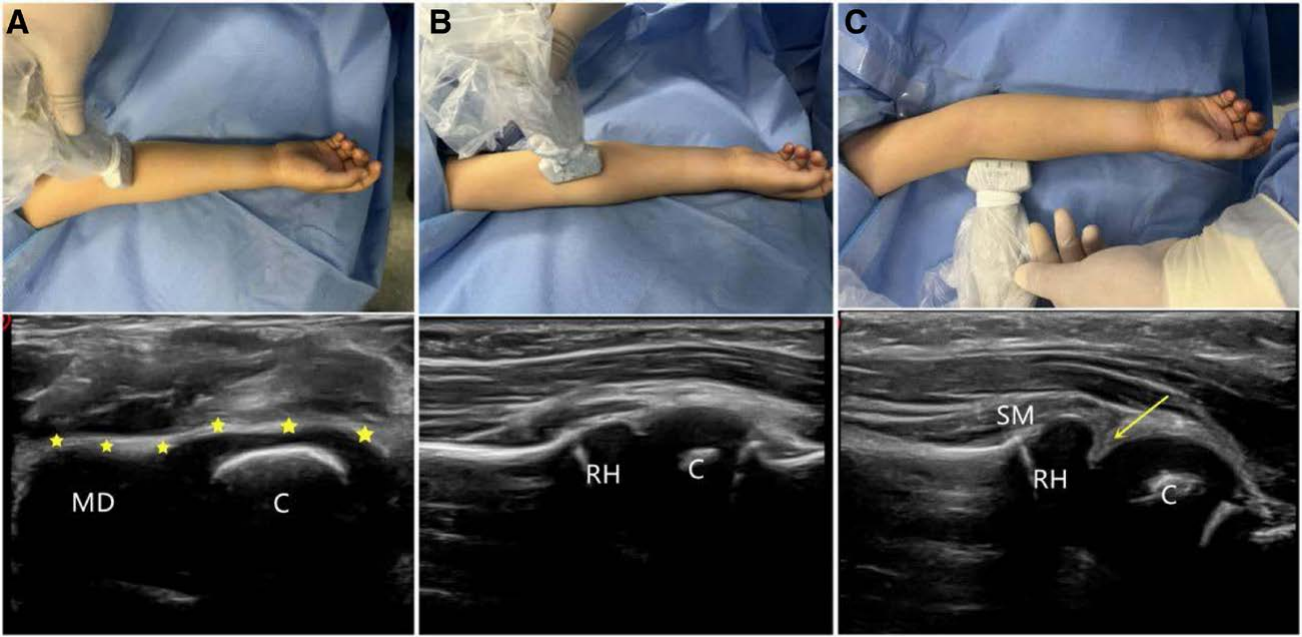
These are pictures of the ultrasound examination positions and mapping related scans of a 4-yr-old boy. (A) On the transverse scan, the normal articular cartilage of distal humeral was clearly showed as a continuous, black, and waving shape (yellow stars). (B) On the coronal longitudinal scan, it showed that there were normal “double-hump sign” and “congruency sign” in radio-capitellum joint. (C) The sagittal longitudinal scan of ultrasound showed that the supinator muscle was advanced and annular ligament was entrapped, indicating a “hook sign” (yellow arrow). C = capitellum; MD = medial condylar; RH = radial head; SM = supinator muscle.
